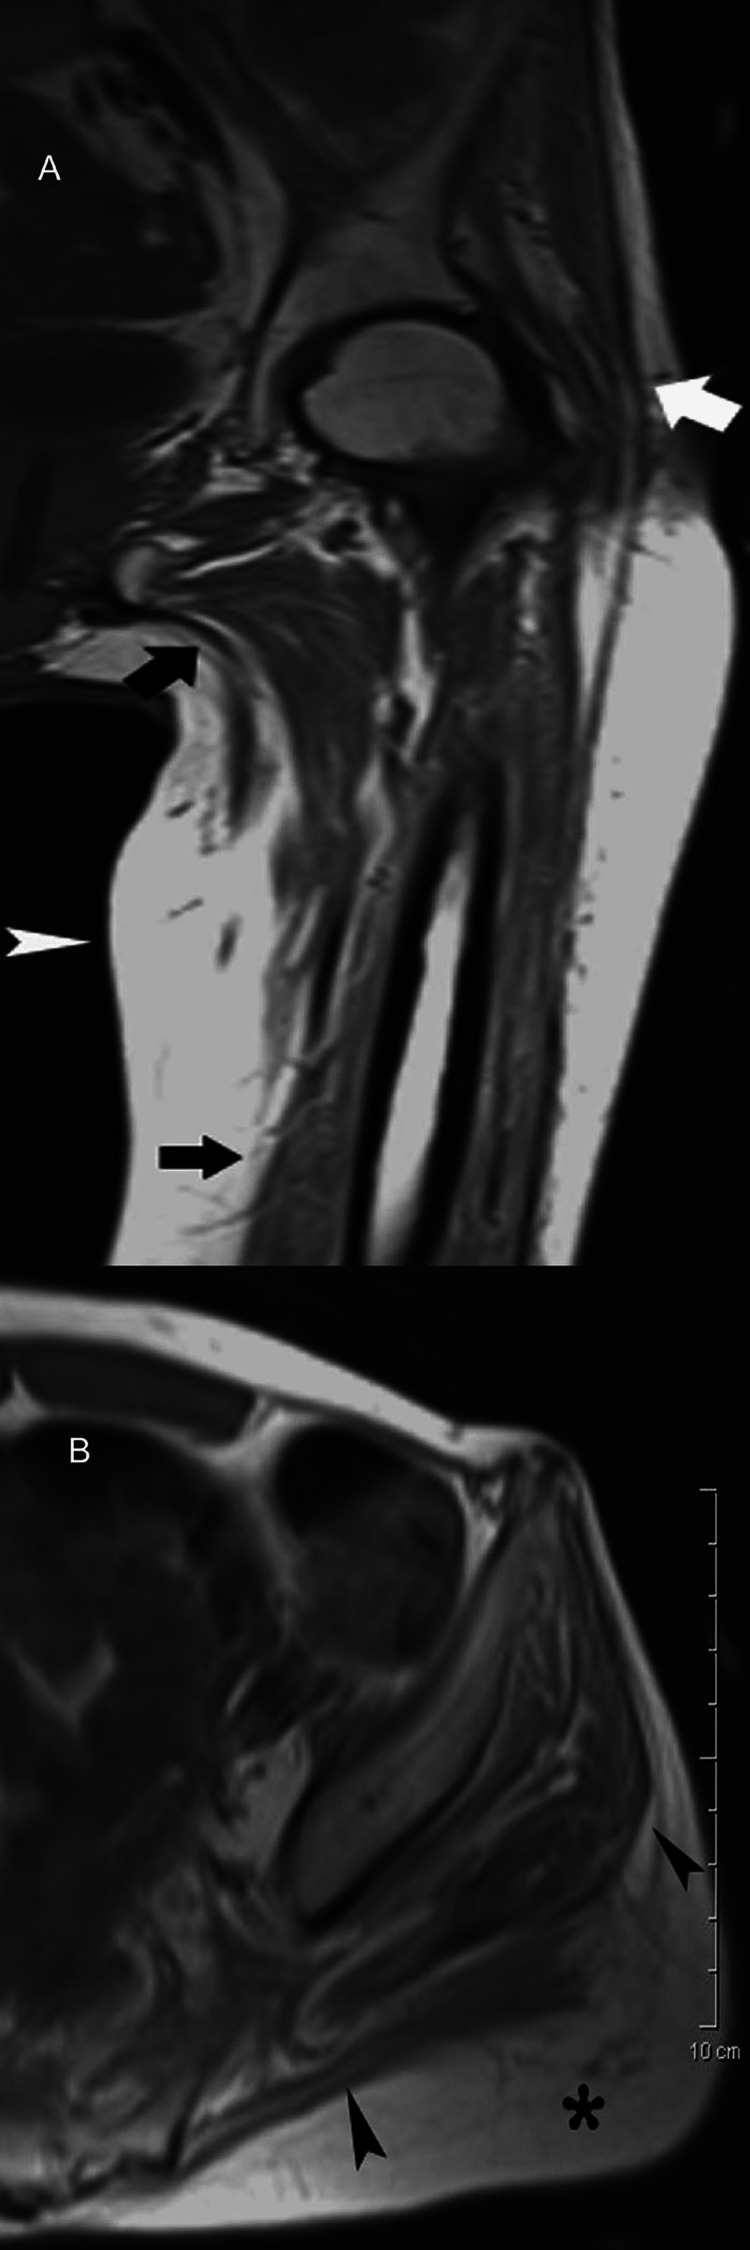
A 34-year-old male with muscle weakness after receiving prolonged treatment with corticosteroids for Crohn’s disease. (A) Coronal T1-weighted MR image reveals overt generalized atrophy of muscles around the hip and thigh (arrows). Note the increased accumulation of fat in the thigh (arrowhead). (B) Corresponding axial T1-weighted MR image shows marked fatty infiltration of the gluteal muscles (arrowheads). The regional fat depot is seen in the buttock (asterisk).
